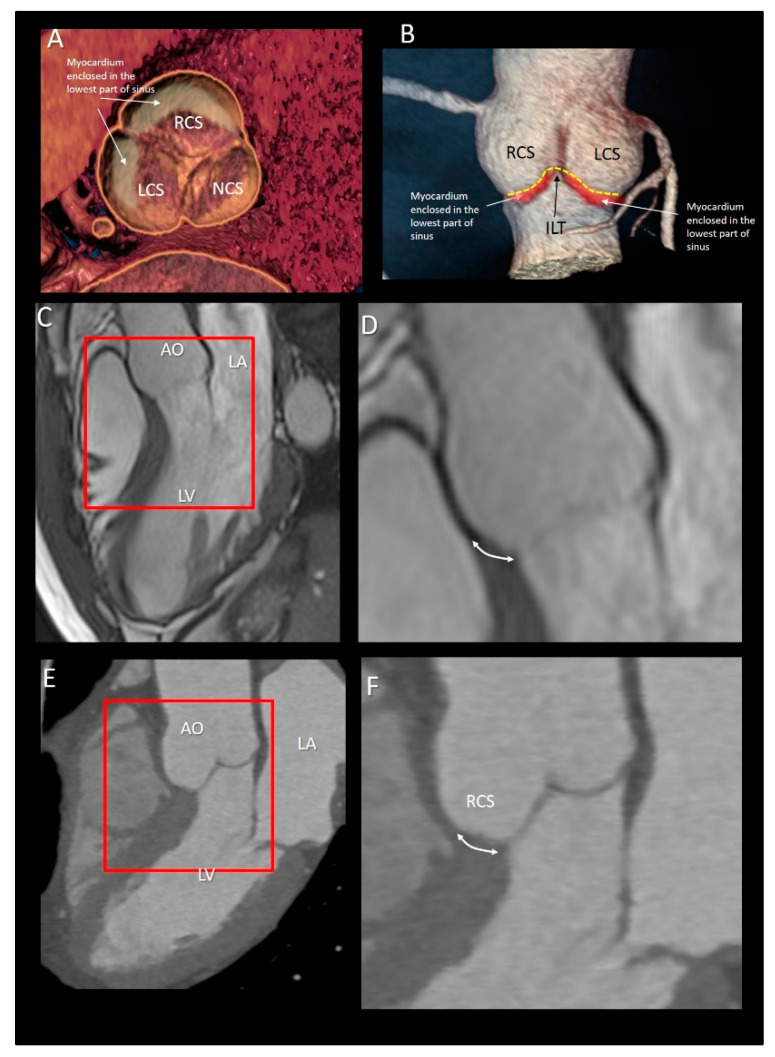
(A) CT image of short-axis view of the aortic root from an aortic perspective. The gray-beige areas superimposed to the image indicate the myocardium enclosed at the bases of right coronary sinus (RCS) and left coronary sinus (LCS). (B) CT scan of volumetric image of the aortic root. The ventriculo–arterial junction is indicated by the yellow dotted line. The red areas below indicate the muscular myocardium enclosed in the bottom part of the sinuses. (C) Cardiac magnetic resonance showing the left ventricle (LV), the left atrium (LA), and the aorta (AO) in cross-section long-axis view. (D) Magnified image of the structures in the red square of the panel C. The double-headed arrow marks the extension of muscular sleeve on the right coronary sinus (RCS). (E) Computed tomography showing the LV, the LA, and the AO in cross section long-axis view. (F) Magnified image of the structures in the red square of the panel C. The double-headed arrow marks the extension of muscular sleeve on the RCS.
